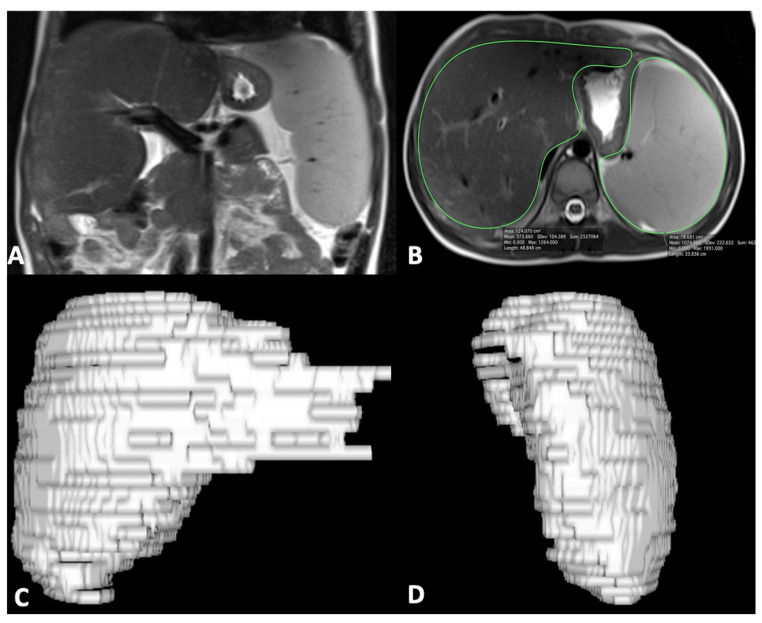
Coronal MR image shows liver and spleen enlargement (A); axial MR image shows ROI analysis of liver and spleen (B) to obtain 3D liver (C) and spleen (D) volume reconstruction images; of note, ROI analysis was performed on multiple sequential slices for completely including the liver and spleen.
